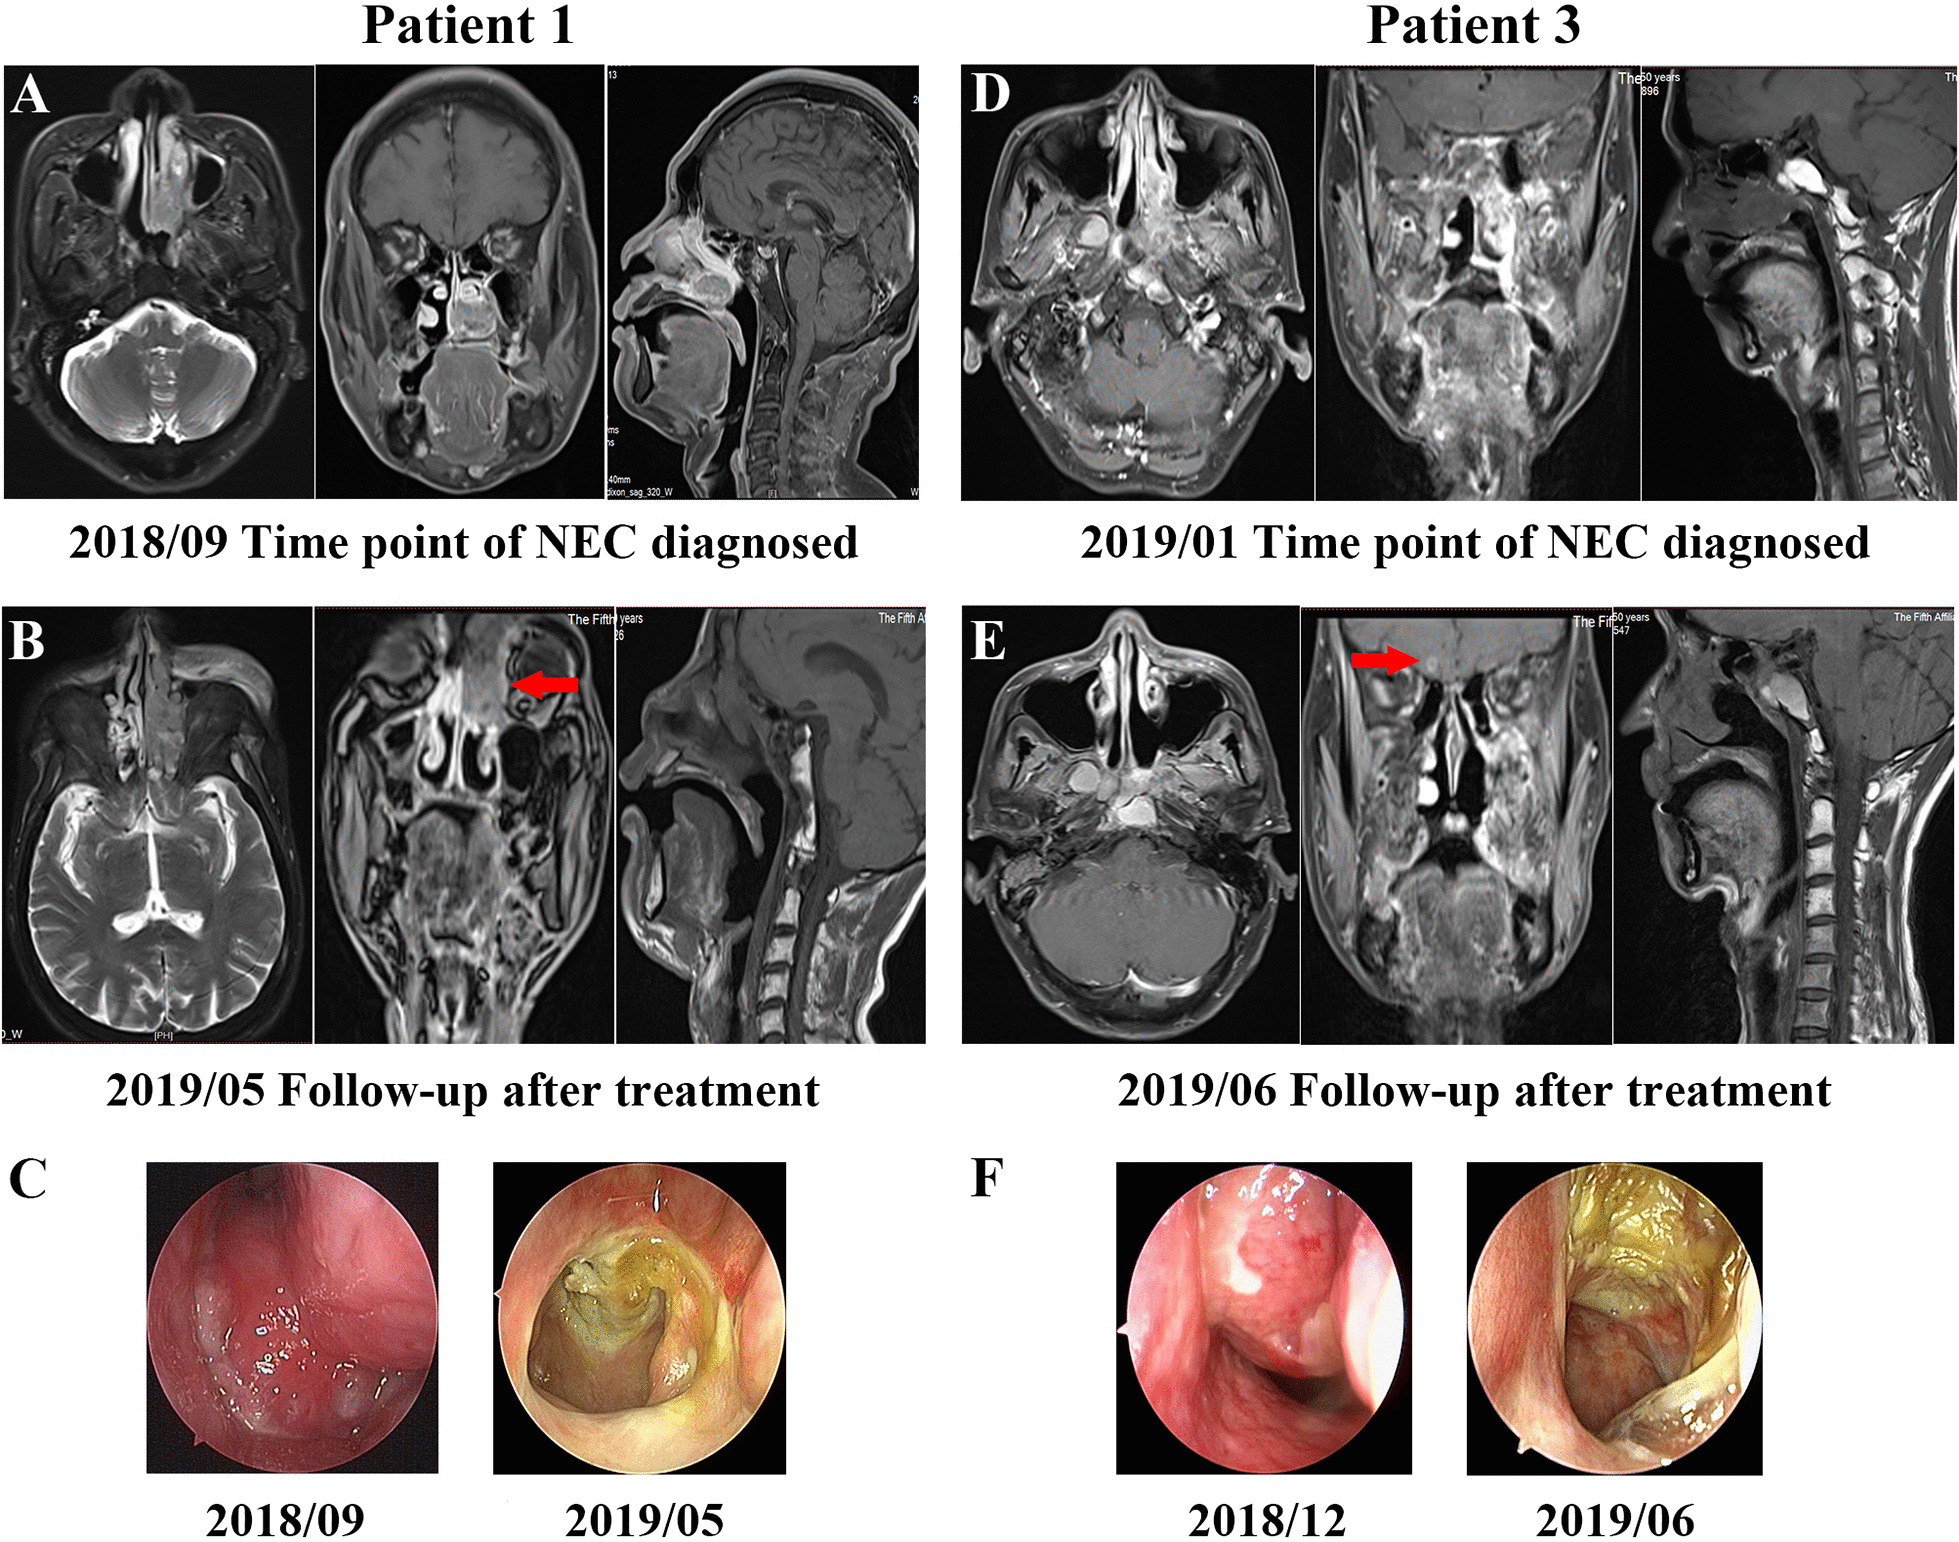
MRI and nasal endoscopy images at diagnosis and during the follow-up of NEC of Patient 1 and Patient 3. A MRI images of patient 1 at diagnosis. B MRI images of Patient 1 during the follow-up. Progression in the upper bound of the tumor during the follow-up of Patient 1 (red arrow). C Nasal endoscopy images at diagnosis (2018/09) and during the follow-up (2019/05) of Patient 1. D MRI images of patient 3 at diagnosis. E MRI images of Patient 3 during the follow-up. Brain metastasis during the follow-up of Patient 3 (red arrow). F Nasal endoscopy images at diagnosis (2018/12) and during the follow-up (2019/06) of Patient 3
